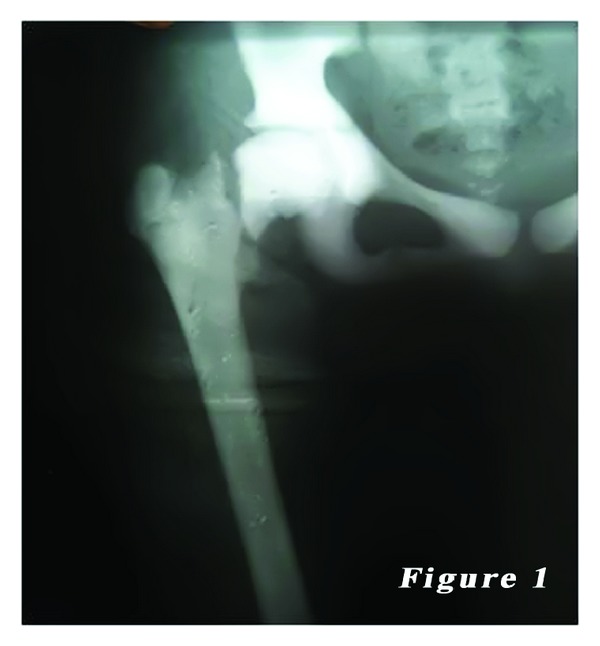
14-year-old female patient (first case) preoperative radiography of the right femoral neck fracture.
